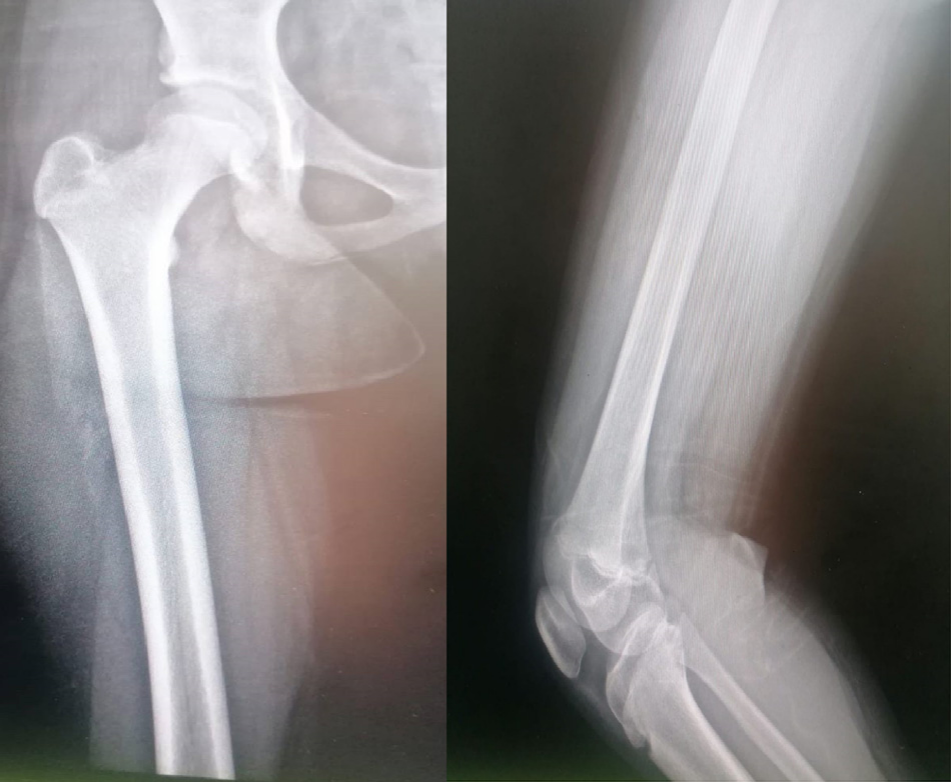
Initial presentation x-ray AP and lateral views showing no obvious pathology.
